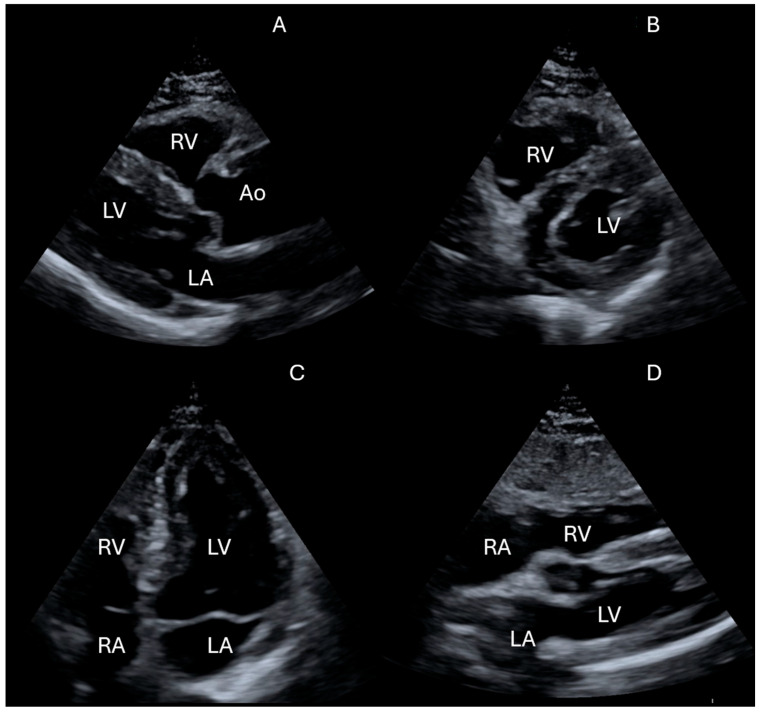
Basic FoCUS views commonly used in bedside echocardiographic assessment. (A) Parasternal long-axis. (B) Parasternal short-axis. (C) Apical 4-chamber. (D) Subcostal 4-chamber. (RV) Right ventricle. (LV) Left ventricle. (RA) Right atrium. (LA) Left atrium. (Ao) Aorta.
